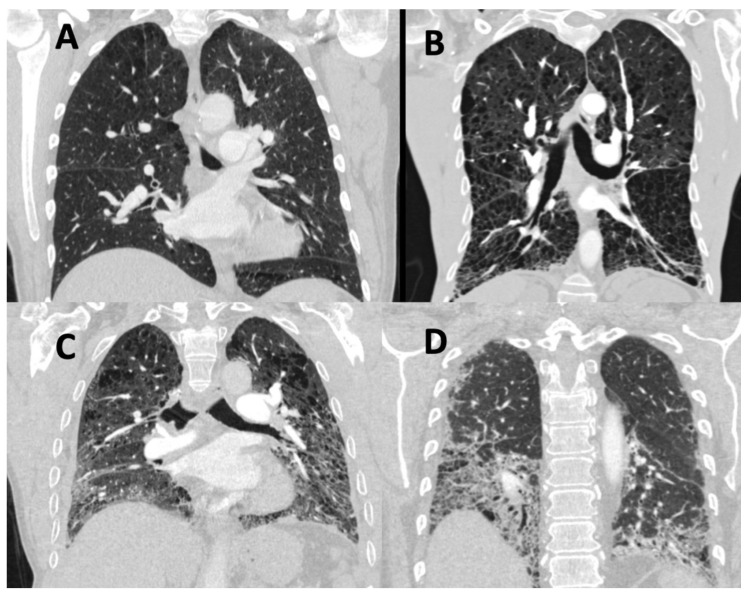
Patterns of lung disease in pulmonary hypertension in association with lung disease and/or hypoxia (PH-Lung) as visualized on CT. (A) Mild emphysema localized predominantly to the upper lobe. (B) Widespread severe emphysema. (C) Combined emphysema and fibrosis. (D) Interstitial lung disease.
